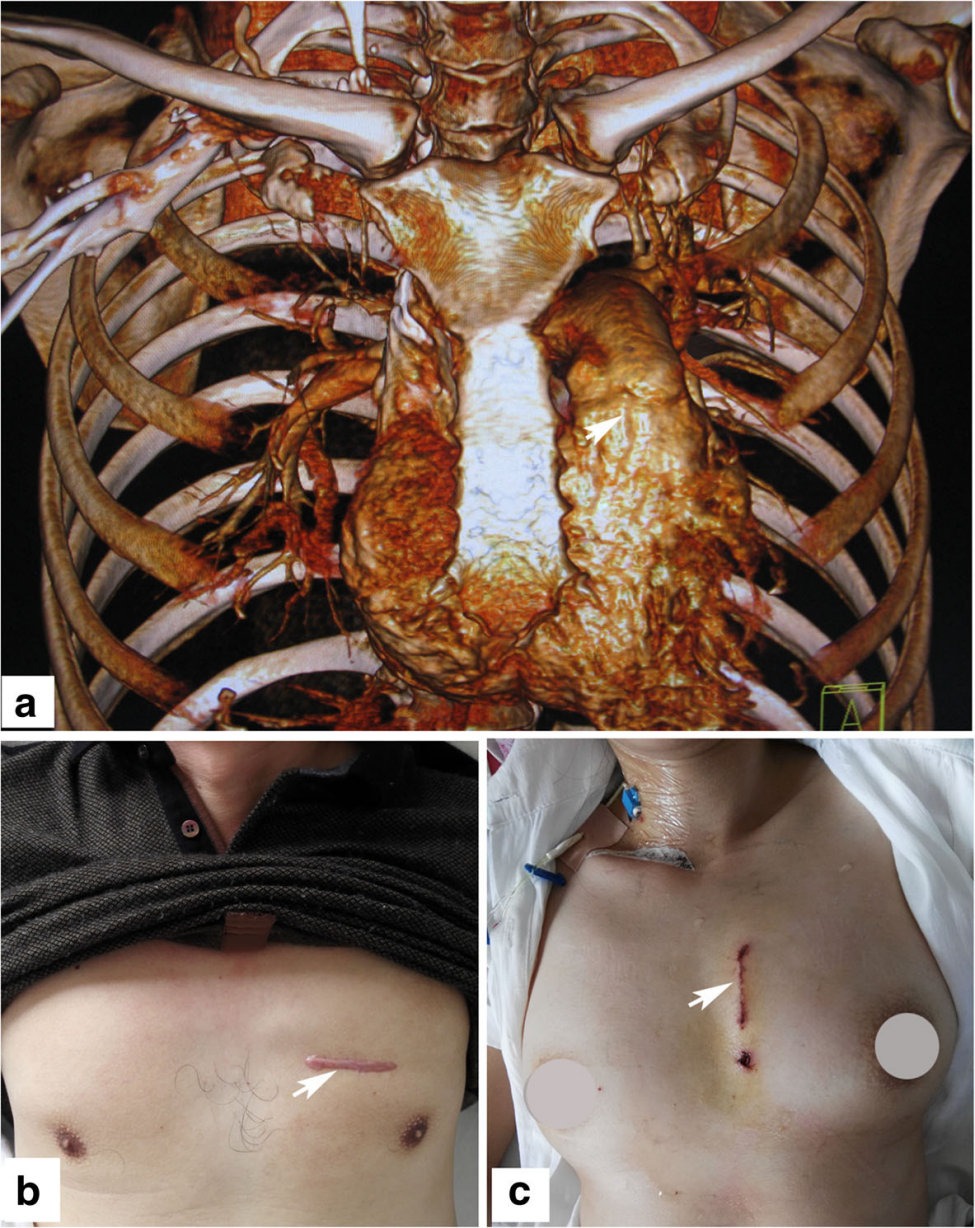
Minimally invasive cardiac surgical technique via left anterior mini-thoracotomy is used for the repair of adult subarterial VSDs. a The chest CT scan combined with 3-dimensional reconstruction is used for positioning the subarterial VSD site (the *white arrowhead* shows). The *arrowheads* indicate the skin incision accesses used for repair of subarterial VSDs via left anterior mini-thoracotomy, b transverse incision was only chosen by male patients, c longitudinal incision was mainly chosen by female patients
